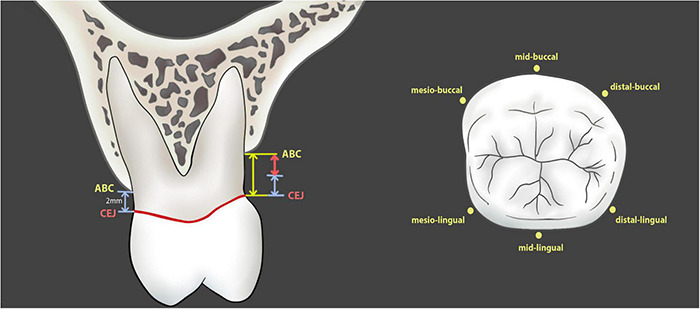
Measurement of alveolar bone loss in patients and in CBCT images. The numerical value of alveolar bone loss is the distance from alveolar bone crest to cementoenamel junction minus 2 mm (red line with double arrow).
